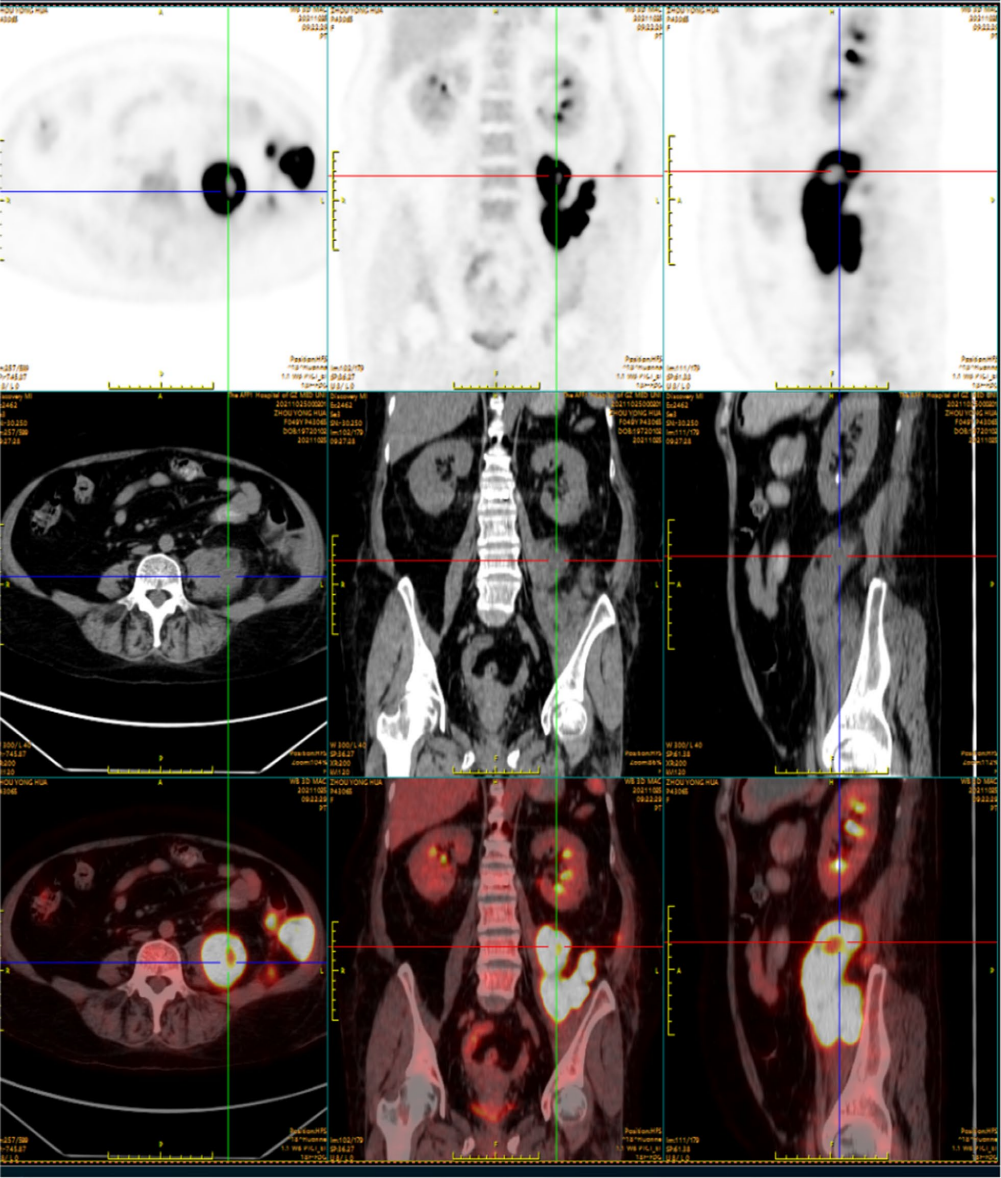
The coronal and sagittal section on abdominal ^18^F-FDG PET/CT. ^18^F-FDG PET, CT on the soft-tissue window, and fused PET/CT images demonstrated FDG accumulation in the left middle and lower abdomen. The hypermetabolic areas (yellow-white) indicated that the FDG-avid soft tissue mass was in an active inflammatory process
